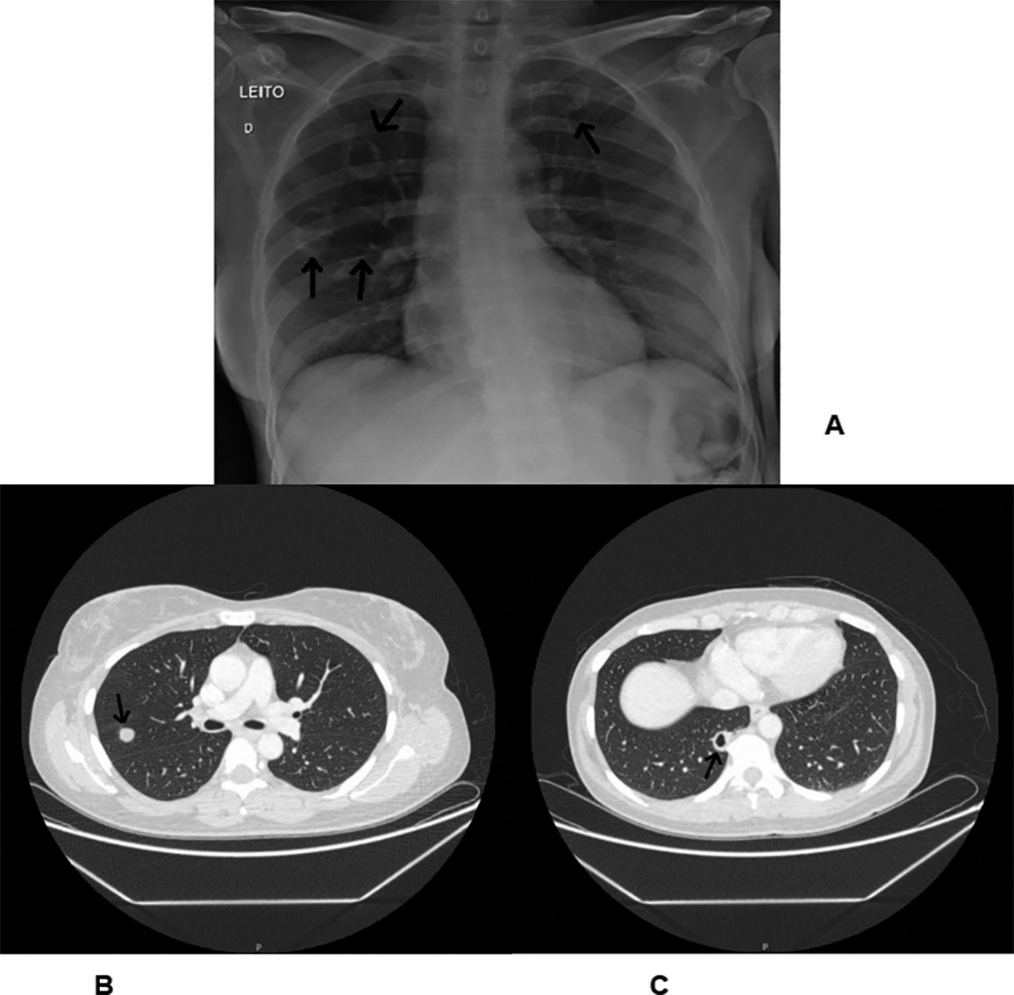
(A) Chest radiography in PA view showing bilateral nodules (patient
